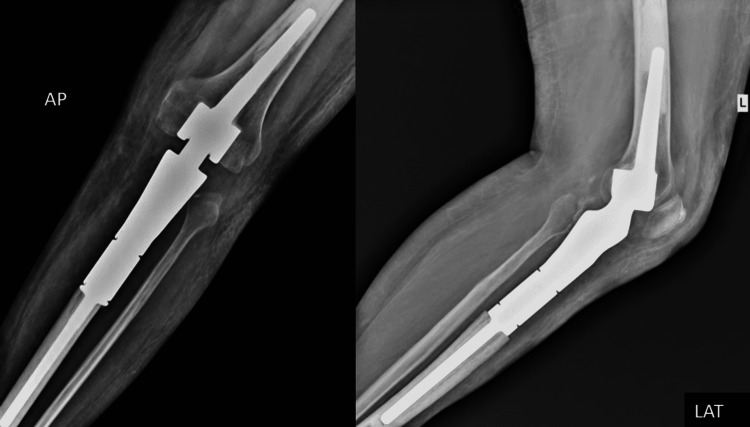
Postoperative x-ray AP and lateral view with mega-prosthesis in-situ AP: anteroposterior
